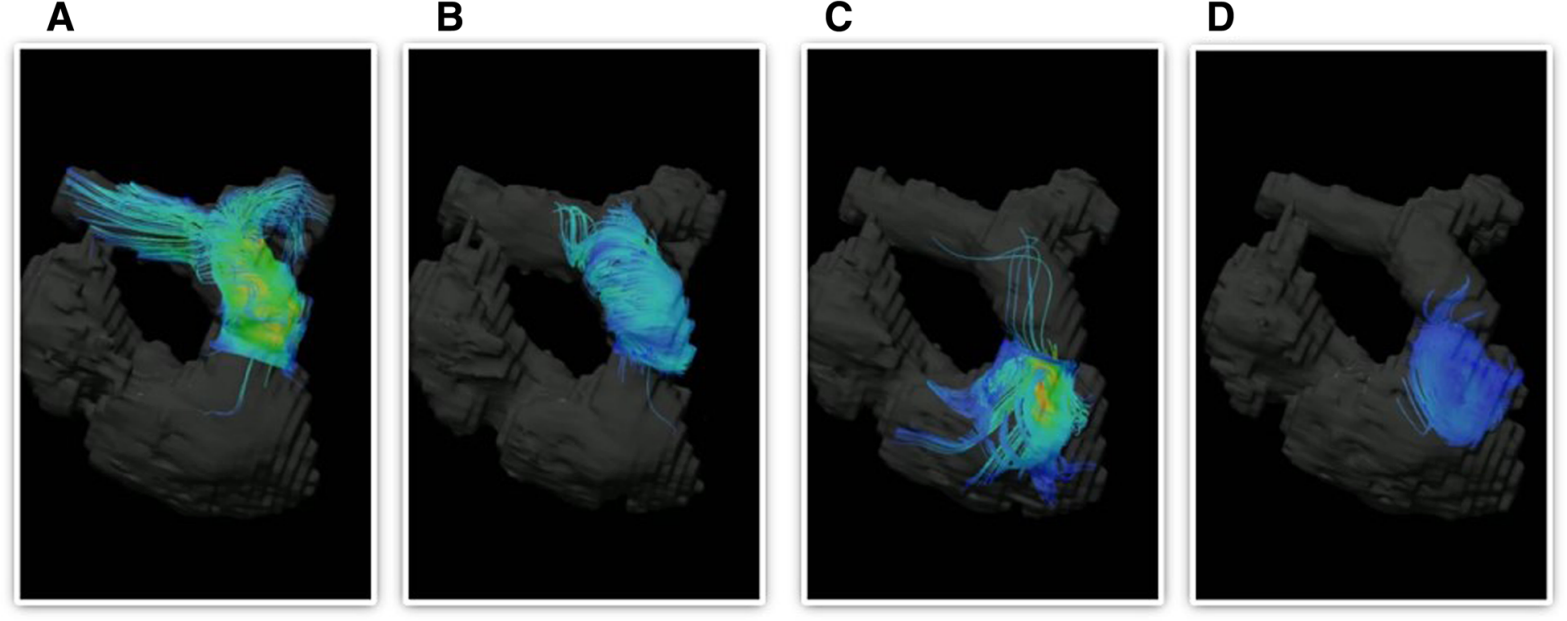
Pulmonary regurgitation of three-dimensional pathline from four-dimensional flow magnetic resonance imaging. a early systolic phase, b late systolic phase, c early diastolic phase, and d late diastolic phase
